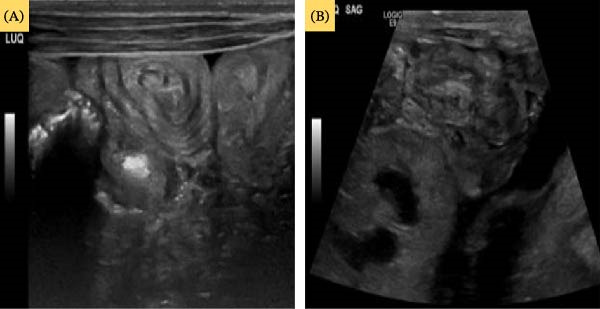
Targeted ultrasound to the right lower abdomen showing (A) target sign and (B) pseudo‐kidney sign, suggestive of intussusception in the terminal ileum (Case 1).
